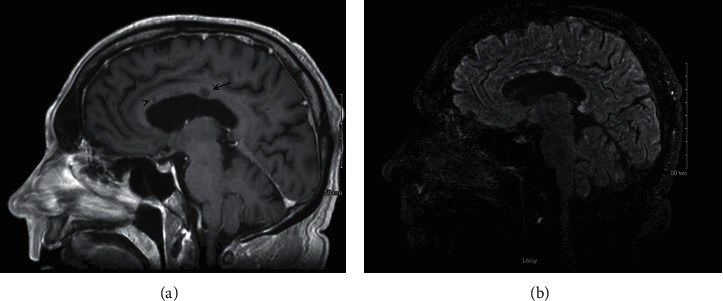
Sagittal views at the level of the corpus callosum during active susac syndrome. (a) T1-weighted. *Note*. The 6-7 mm T1 hypodensity in the body of the corpus callosum was consistent with a “snowball” lesion (demarcated by an arrow), and T1 linear hypodensity sparing the calloseptal surface of the corpus callosum was consistent with a linear “spoke” or “icicle” lesion (demarcated by an arrow head). (b) Sagittal T2/FLAIR-weighted imaging with gadolinium contrast. *Note*. The increased T2/FLAIR signal and contrast enhancement in the “snowball” lesion (demarcated by an arrow) were consistent with active susac syndrome.
